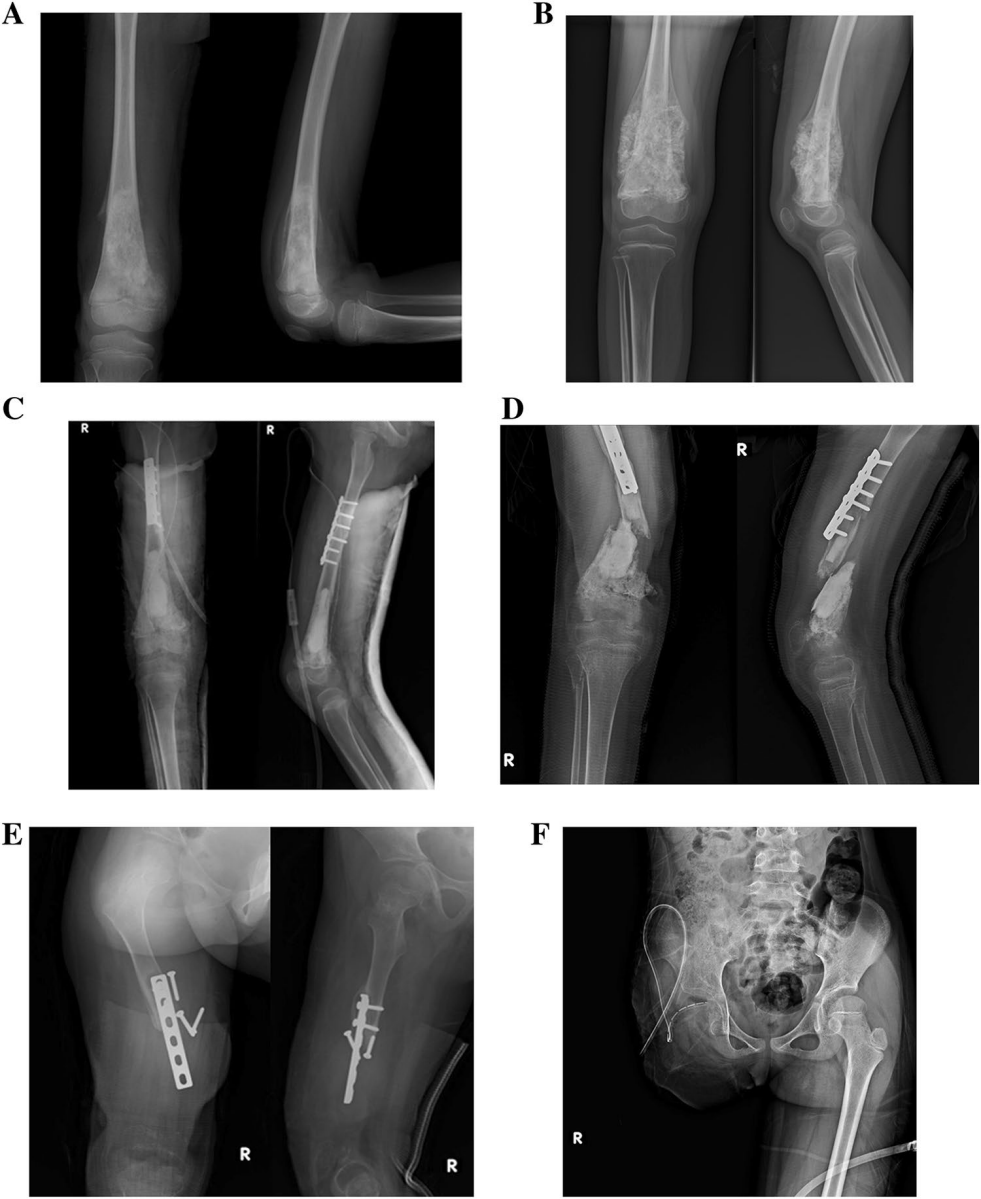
A 6-year-old girl with conventional osteosarcoma of the right distal femur. (A) Preoperative anteroposterior and lateral radiograph. (B) After chemotherapy, an osteoblastic lesion of the distal femur was observed. (C) Wide resection and reconstruction with recycled frozen autograft were performed. (D) Fracture of the graft occurred 1 year after surgery. (E) Lysis of the graft was found 15 months later. (F) Finally, hip disarticulation was performed.
